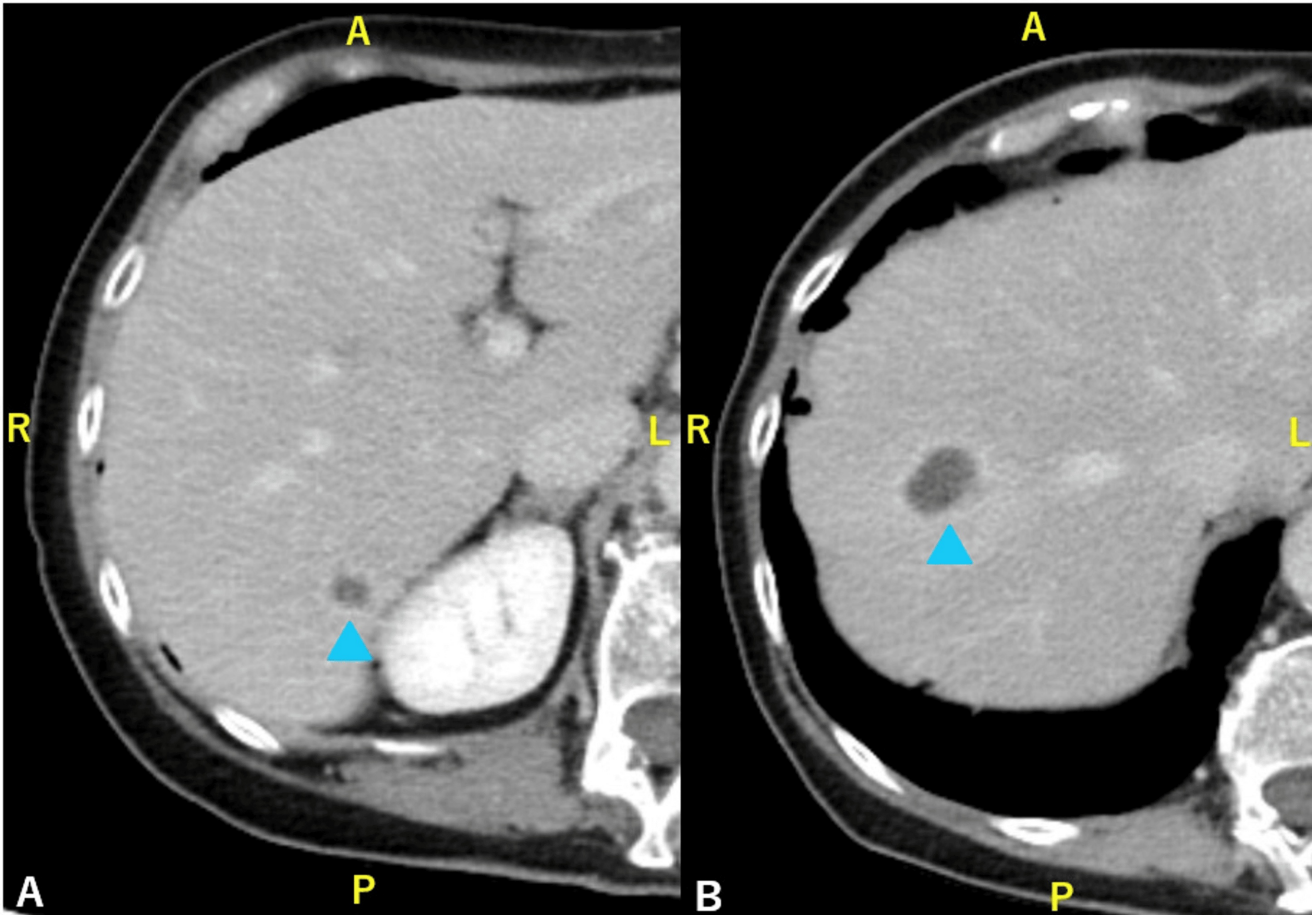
Postoperative contrast-enhanced CT on day six after laparoscopic ileocecal resection (A, B) Marked regression of the hepatic abscesses is evident (arrowheads) CT: computed tomography
